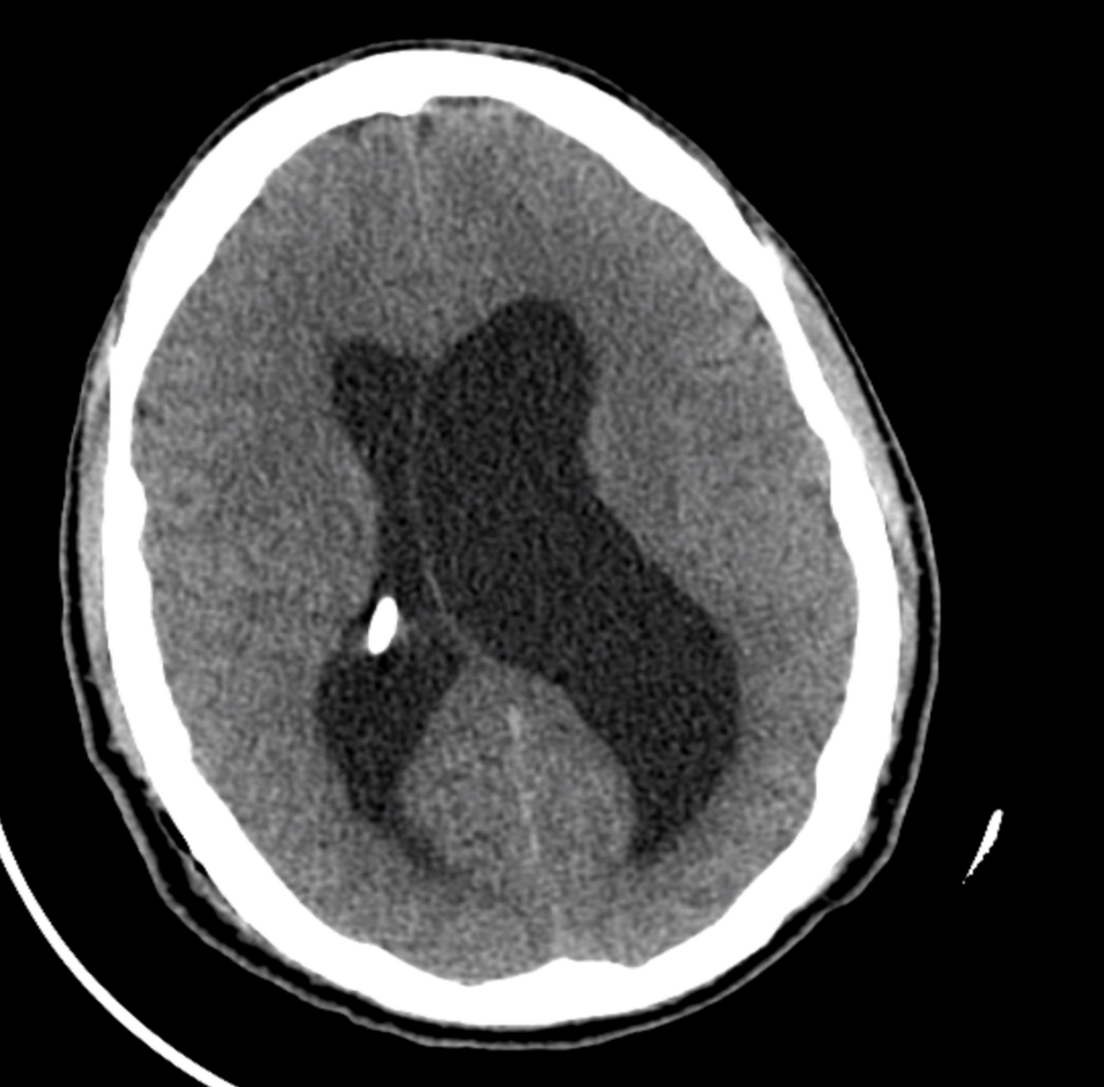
Postoperative axial CT following right ventriculoperitoneal shunt placement, showing selective decompression of the right ventricle and rightward midline shift, consistent with left ventricular isolation.
